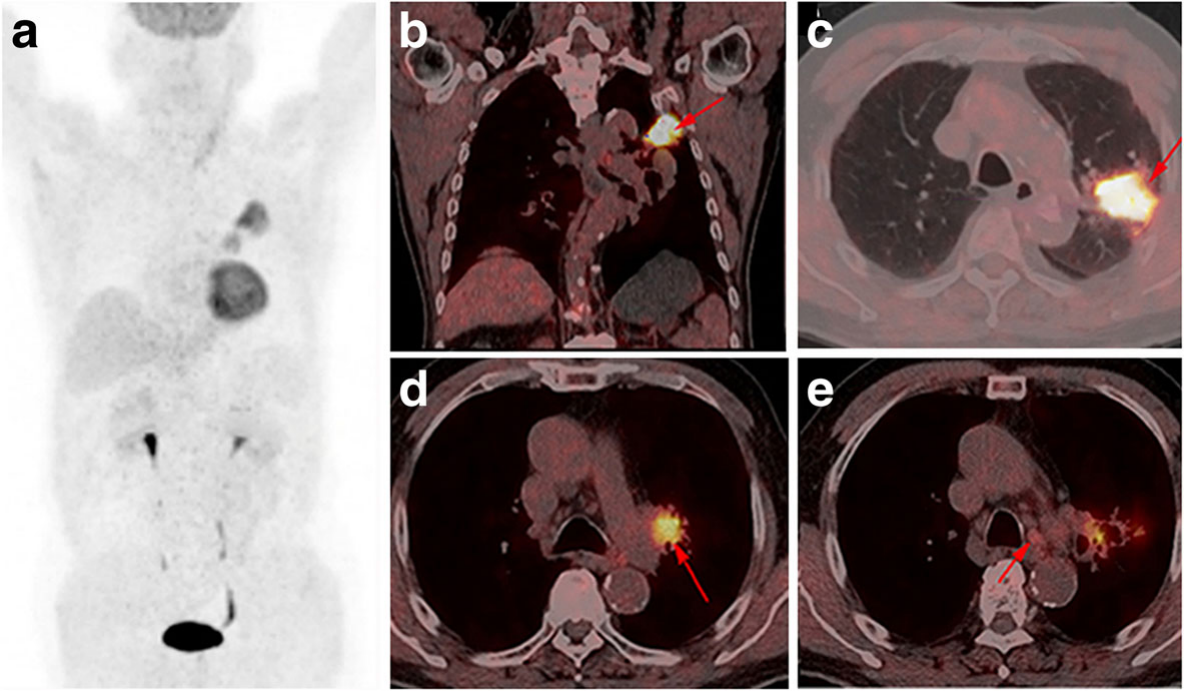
a A positron emission tomography-computed tomography scan shows two hypermetabolic lesions in the upper lobe of the left lung and ipsilateral hilar lymph node. b-c Axial view of positron emission tomography-computed tomography scans for hypermetabolic lesion in the upper lobe of his left lung (*red arrows*), d in the left hilar lymph node (*red arrow*) and e in the left mediastinal lymph node (*red arrow*)
